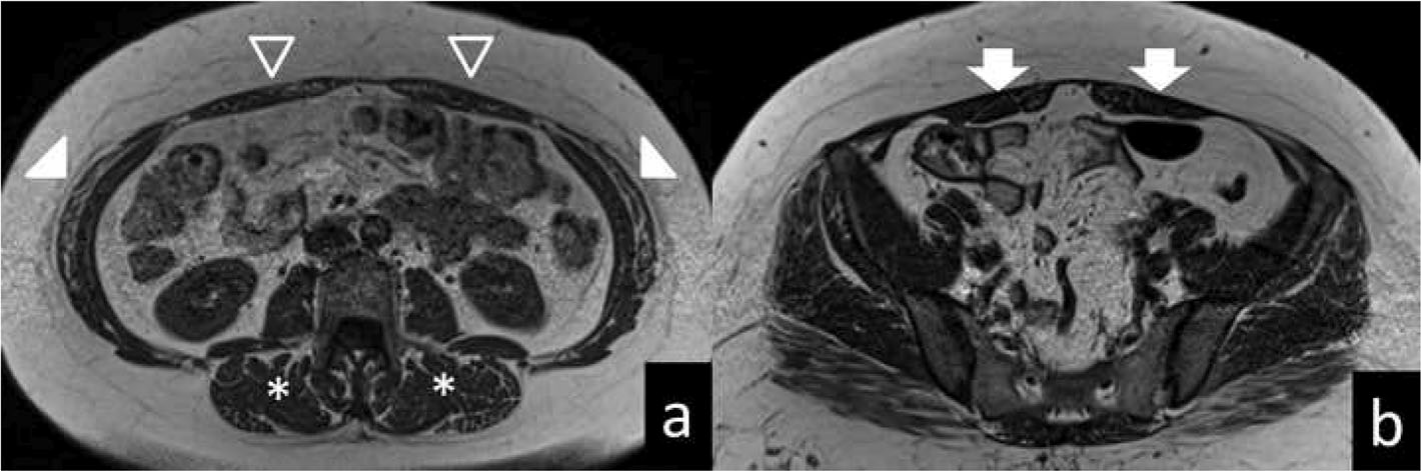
MRI of case. 40-year-old DM1 patient. Transversal T1-weighted sequence in L3/L4- (a) and S1/S2-level (b) show fat infiltration grade 3 in the cranial rectus abdominis (open arrow head) and the external oblique’ s (arrow head), and fat infiltration grade 2 in the caudal rectus abdominis (arrow) and in the erector spinae (*)
